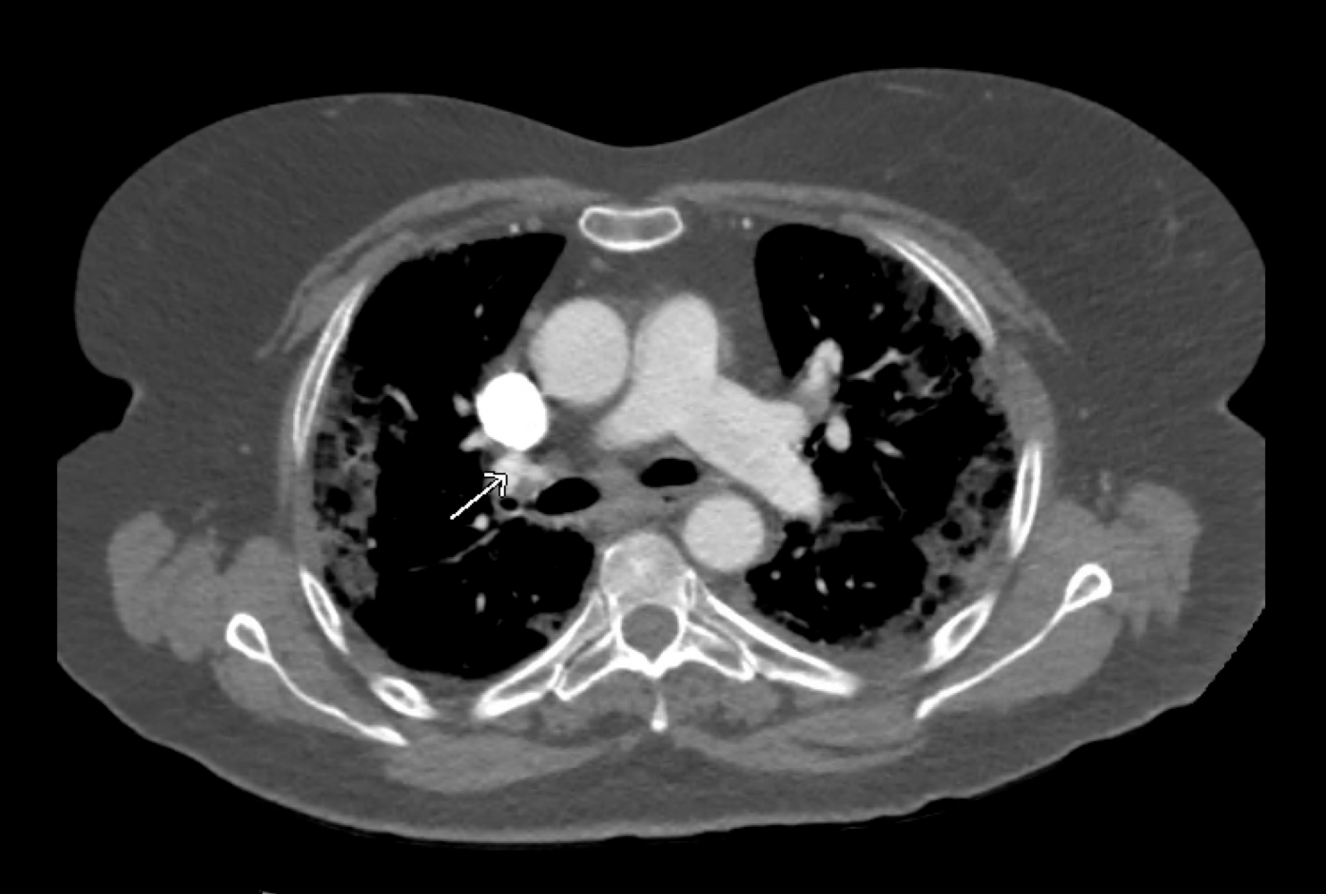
CT angiography of chest showing pulmonary emboli in right upper lobe pulmonary artery and segmental branches of right lower lobe pulmonary artery.
